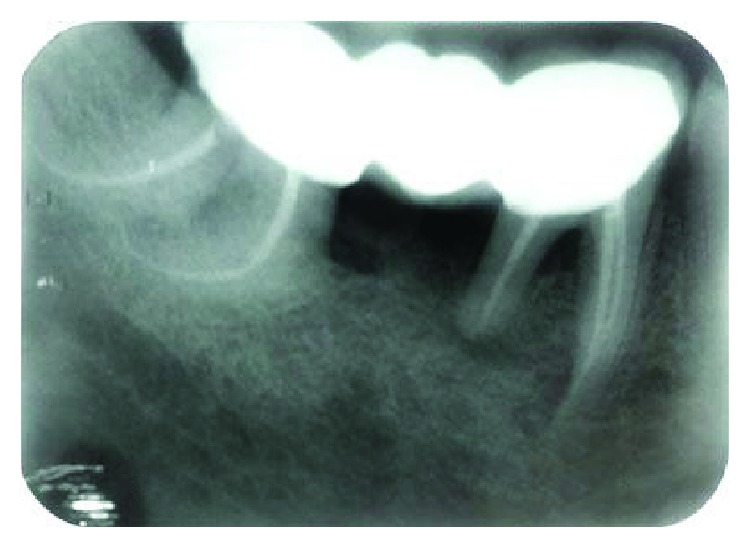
Radiograph showing final restoration.
